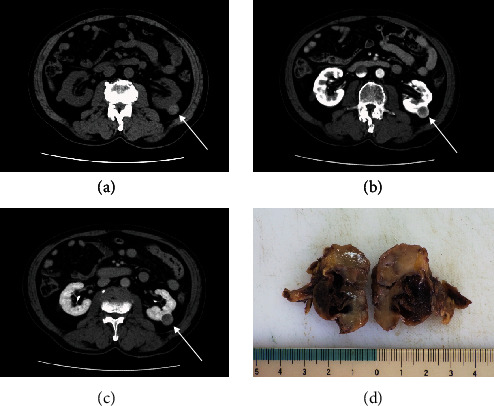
(a) Plain CT: tumor showed slightly dense signal (white arrow). (b) Contrast-enhanced CT: well-defined solid mass was enhanced around the tumor in the early phase (white arrow). (c) Contrast-enhanced CT: tumor was washed out in the late phase (white arrow). (d) Cut surface of the tumor. cystic area was observed inside the tumor.
